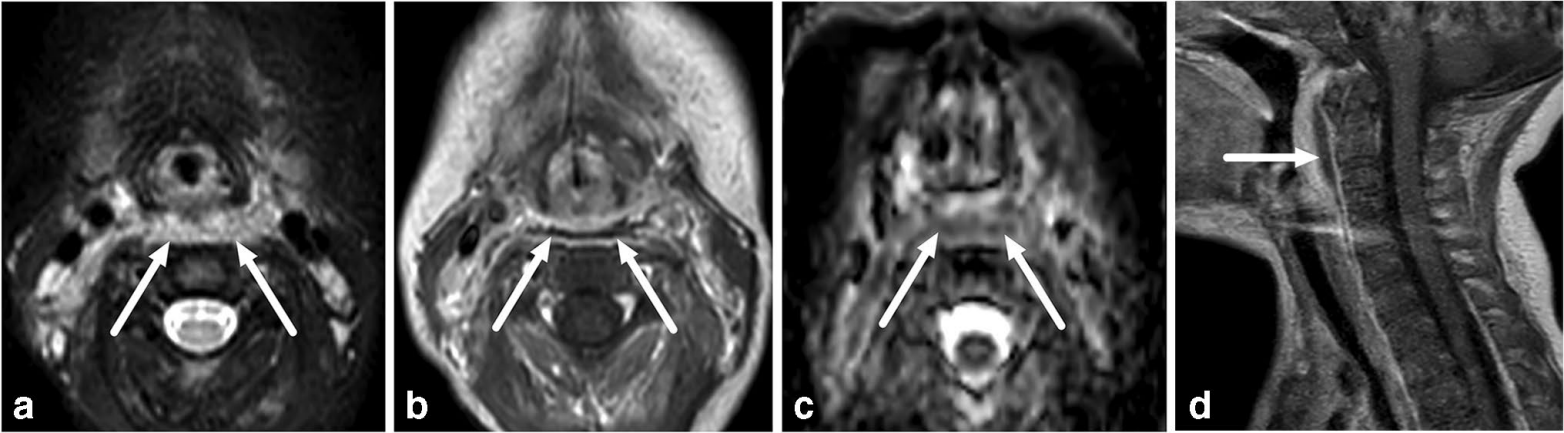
True retropharyngeal abscesses in a 10-month-old boy with a sore throat, who had a thin abscess (*arrows*) that continued caudally into the mediastinum. a Axial T2-weighted with fat saturation. b Axial T1-weighted post-contrast. c Axial apparent diffusion coefficient. d Sagittal T1-weighted post-contrast
